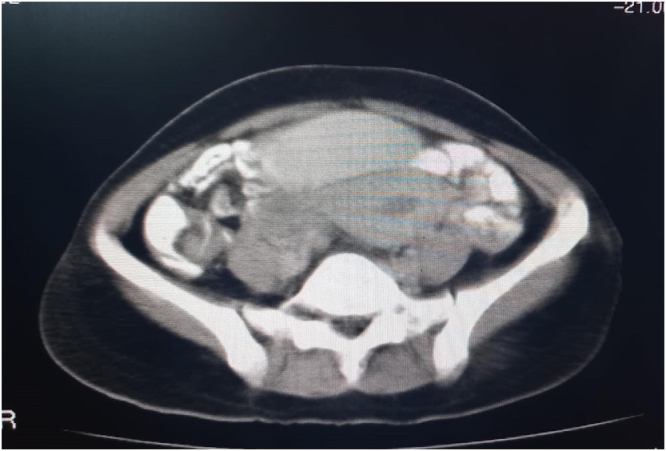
CT Scan of the pelvis showing a large, heterogeneous soft-tissue mass.
